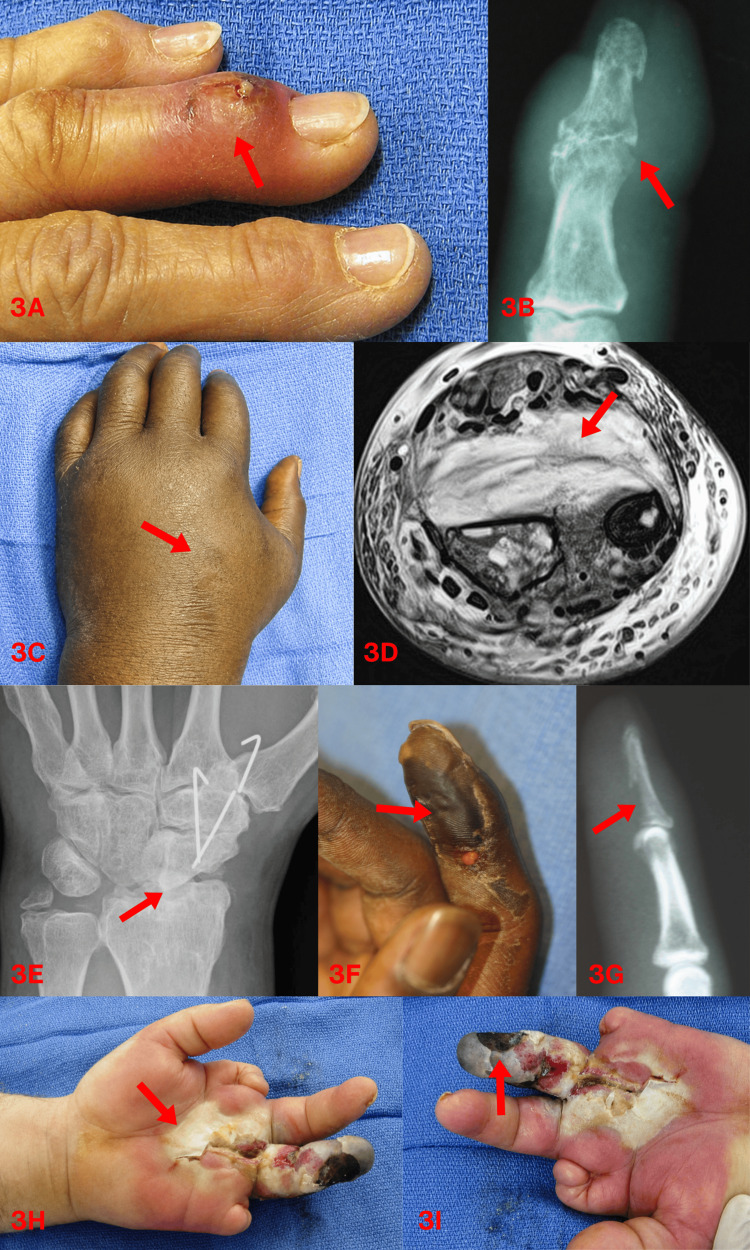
Articular and osseous infections (A-B) Septic arthritis of the finger: gross examination (A) shows swelling and erythema (red arrow) along the distal interphalangeal joint after striking a table; anteroposterior radiograph (B) shows cortical irregularity and joint space narrowing (red arrow). (C-E) Septic arthritis of the wrist: gross examination (C) shows dorsal hand swelling and erythema (red arrow) with limited range of motion; axial MRI (D) reveals joint effusion and synovial enhancement (red arrow), consistent with septic arthritis and early osteomyelitis; anteroposterior radiograph (E) shows joint space narrowing and soft tissue swelling (red arrow) of the radiocarpal joint. (F-G) Osteomyelitis of the distal phalanx: gross examination (F) shows proximal digital swelling, medial skin tension, and distal necrotic changes (red arrow); anteroposterior radiograph (G) reveals cortical erosion and bone lucency (red arrow) at the volar surface of the distal phalanx. (H-I) Gangrene secondary to uncontrolled DM, with palmar ulcerations and erosions (red arrow, H), black necrotic changes in the digits (red arrow, I), and evidence of prior surgical amputation of the index and small fingers. Image courtesy of Gary Schwartz, MD. Used with permission. DM: diabetes mellitus; MRI: magnetic resonance imaging.
